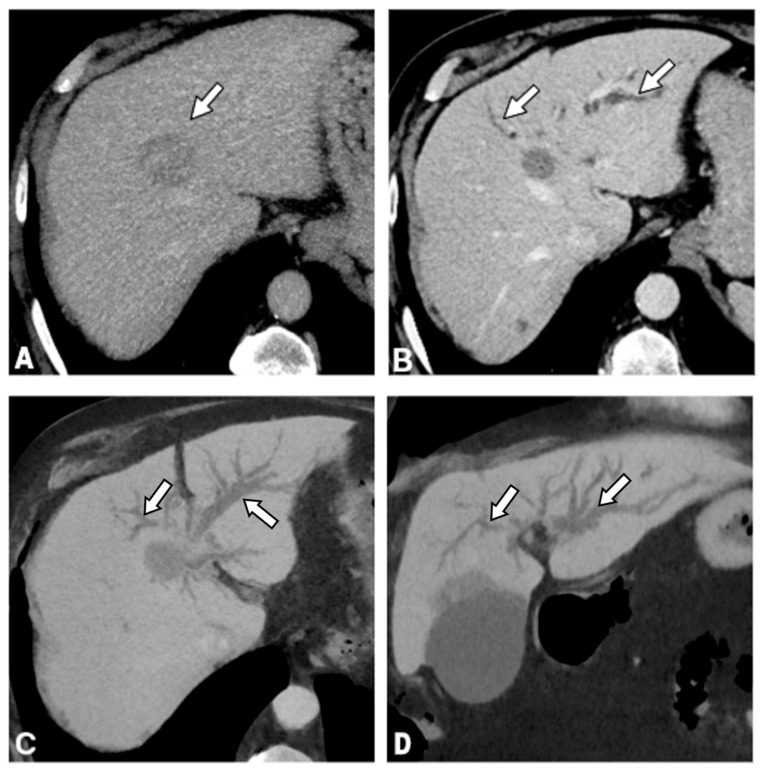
Bile duct dilatation after TACE in a patient, No. 2 from Table 1, with HCC. (A) Baseline contrast-enhanced CT shows HCC (arrow) in segment 4A adjacent to the left hepatic duct. (B) CT obtained 3 months after TACE demonstrates complete tumor necrosis, consistent with complete response, and newly developed diffuse bile duct dilatation (arrows) in the left hepatic lobe. (C,D) MinIP CT reconstructions better depict the dilated bile ducts (arrows) in the left hepatic lobe on axial (C) and coronal (D) images.
